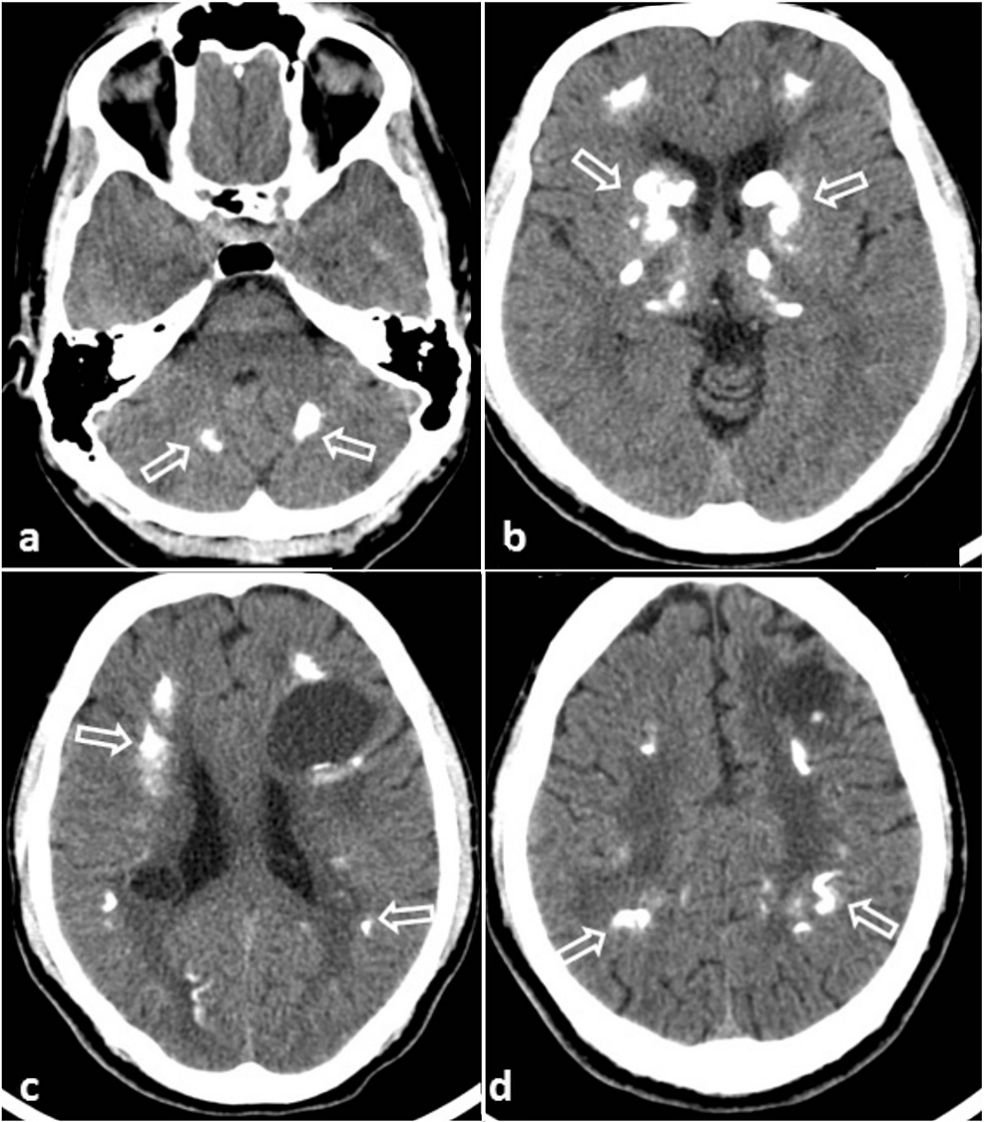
Axial sections of CT brain showing multiple calcific foci in bilateral dentate nuclei (a), basal ganglia (b), and bilateral cerebral white matter (c and d). Also, note the white matter cysts and diffuse white matter hypodensity.
